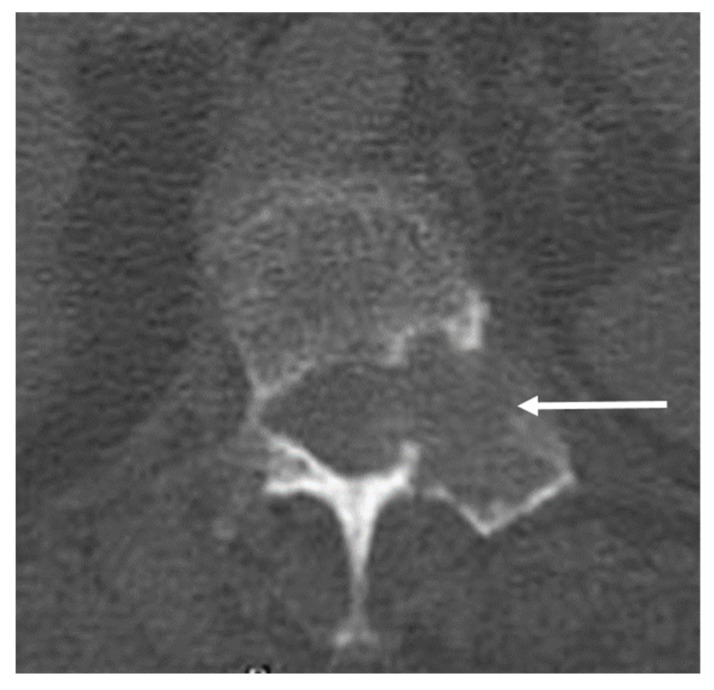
Axial CT showing an aggressive haemangioma of a thoracic vertebra (white arrow). Note the lytic appearance.
